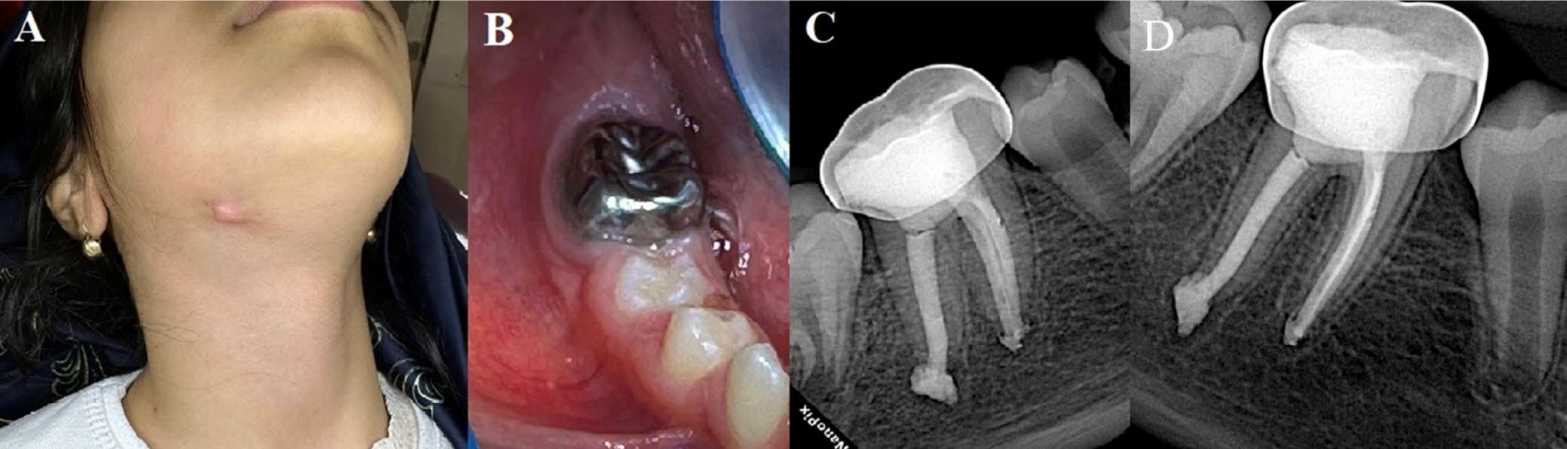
(A) Clinical follow‐up revealed healing of the extraoral sinus tract with residual scar tissue and (B) a functional tooth with healthy gingival tissues. (C) A 6‐month and (D) 12‐month follow‐up radiograph demonstrated substantial reduction of the periapical radiolucency and reestablishment of a normal periodontal ligament space around the roots.
